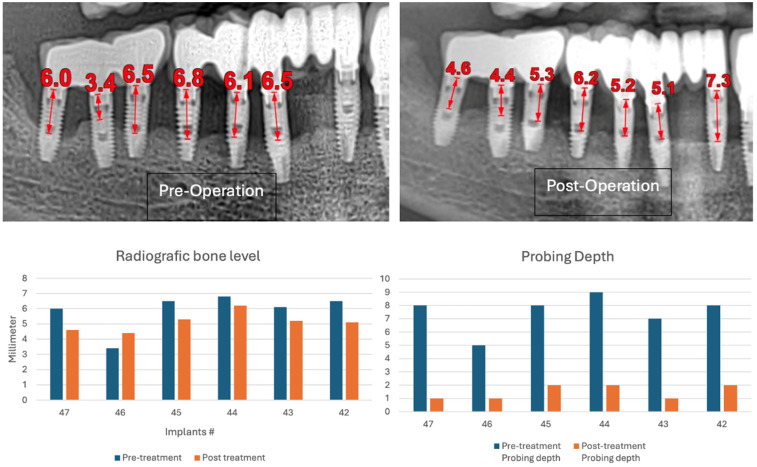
Radiographic bone level and probing depth comparing the baseline and 12-month results.
